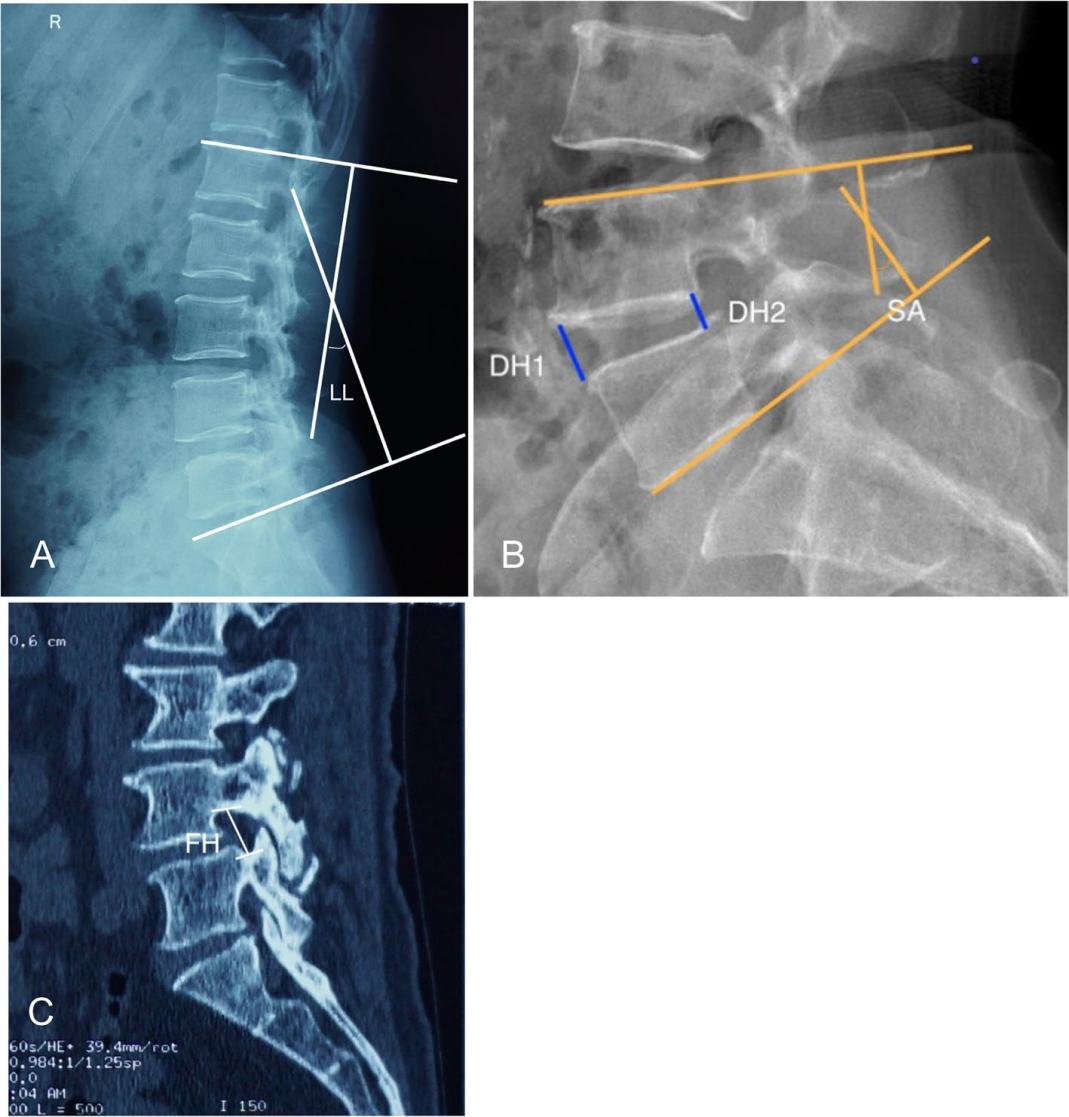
Radiological measurements of LL(A), DH(B), and SA(B) on lateral X-ray. FH was measured on parasagittal reconstruction CT(C). LL, lumbar lordosis; DH, disc height; SA, segmental angle; FH, foraminal height
